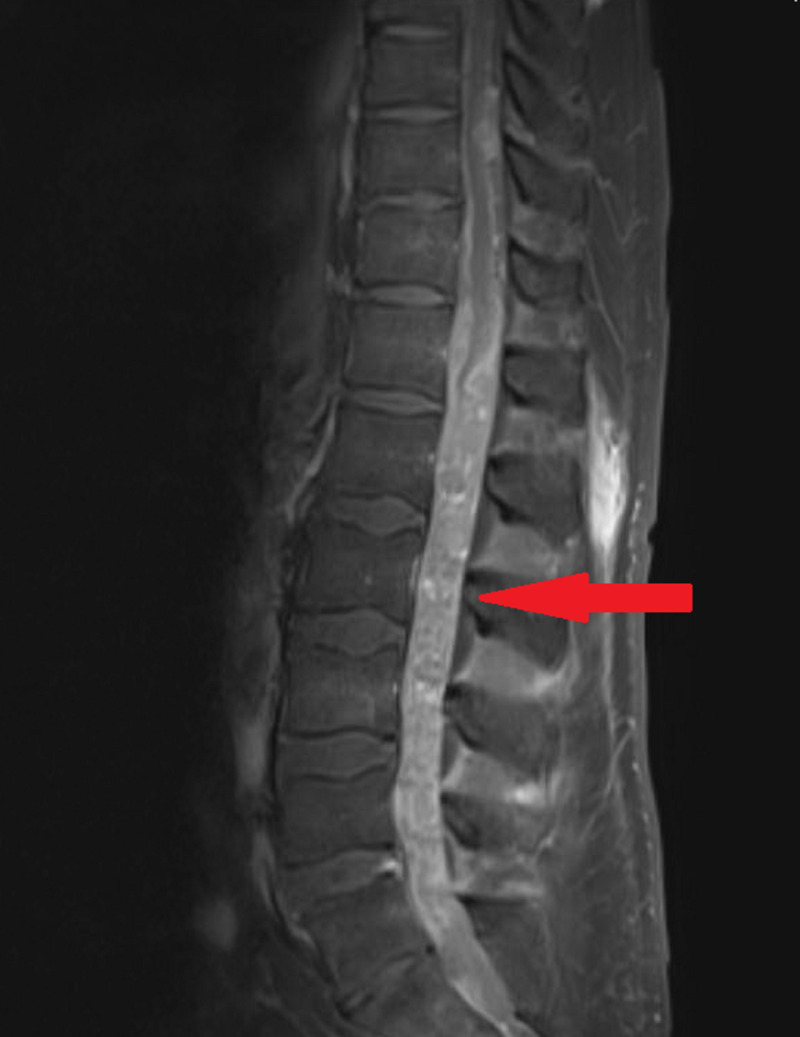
MRI spine (T1-sagittal) showing leptomeningeal enhancement along the lower thoracic and lumbar cord (red arrow)
